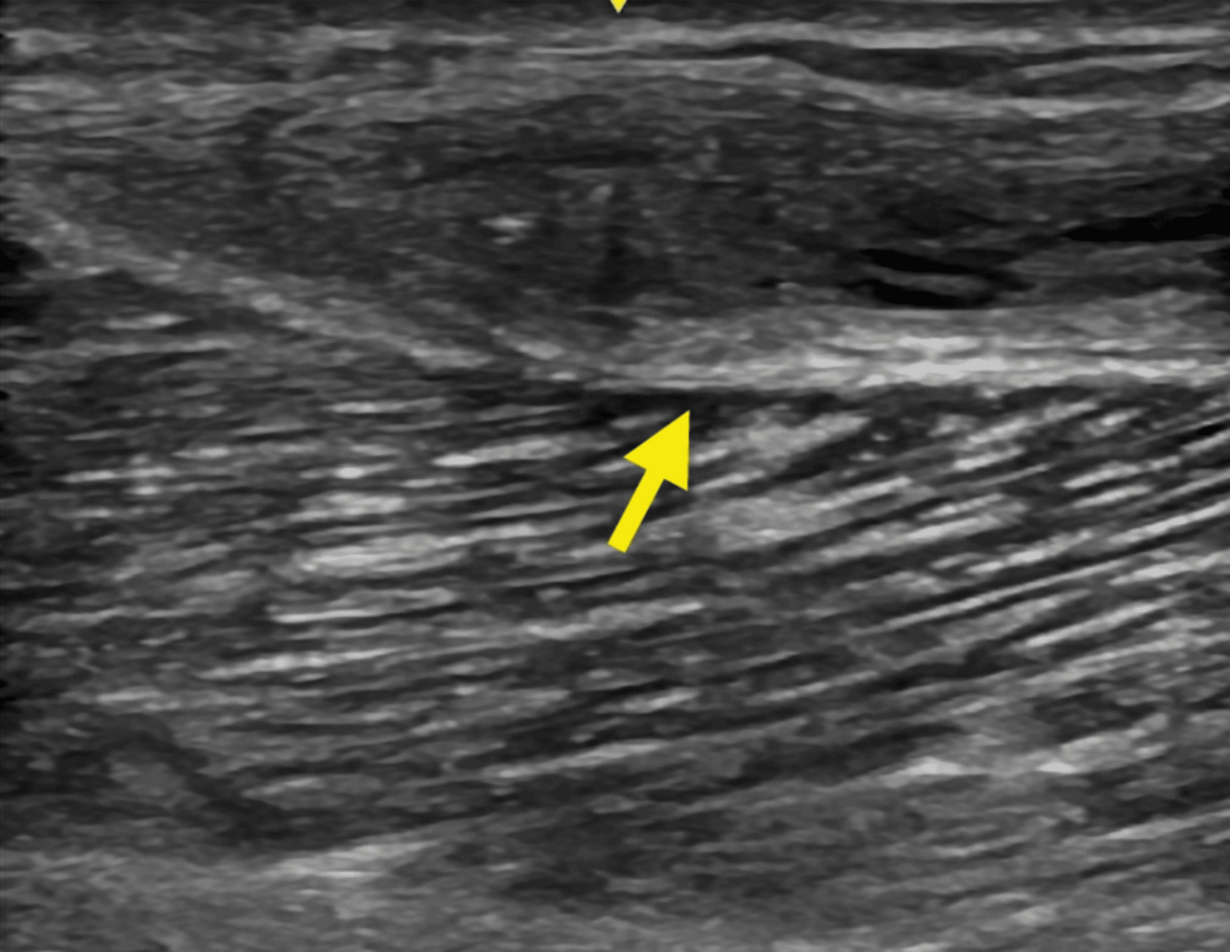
Sonographic image demonstrating sagittal / long axis view of the Baker's cyst status post doxycycline sclerosis demonstrating hyperechoic irregular synovial tissue within the cyst walls which was minimally compressible.
